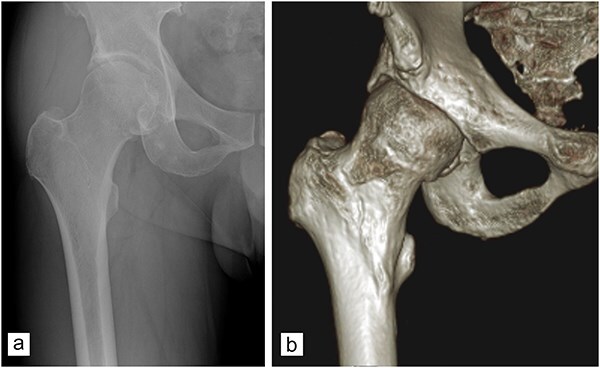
Images from a 68-year-old man with mixed-type FAI. (a) Plain film (AP view) showing a bony structure near the acetabular rim and (b) anterior view of 3D computed tomography reformation showing acetabular overcoverage and bony hump at the femoral head–neck junction.
